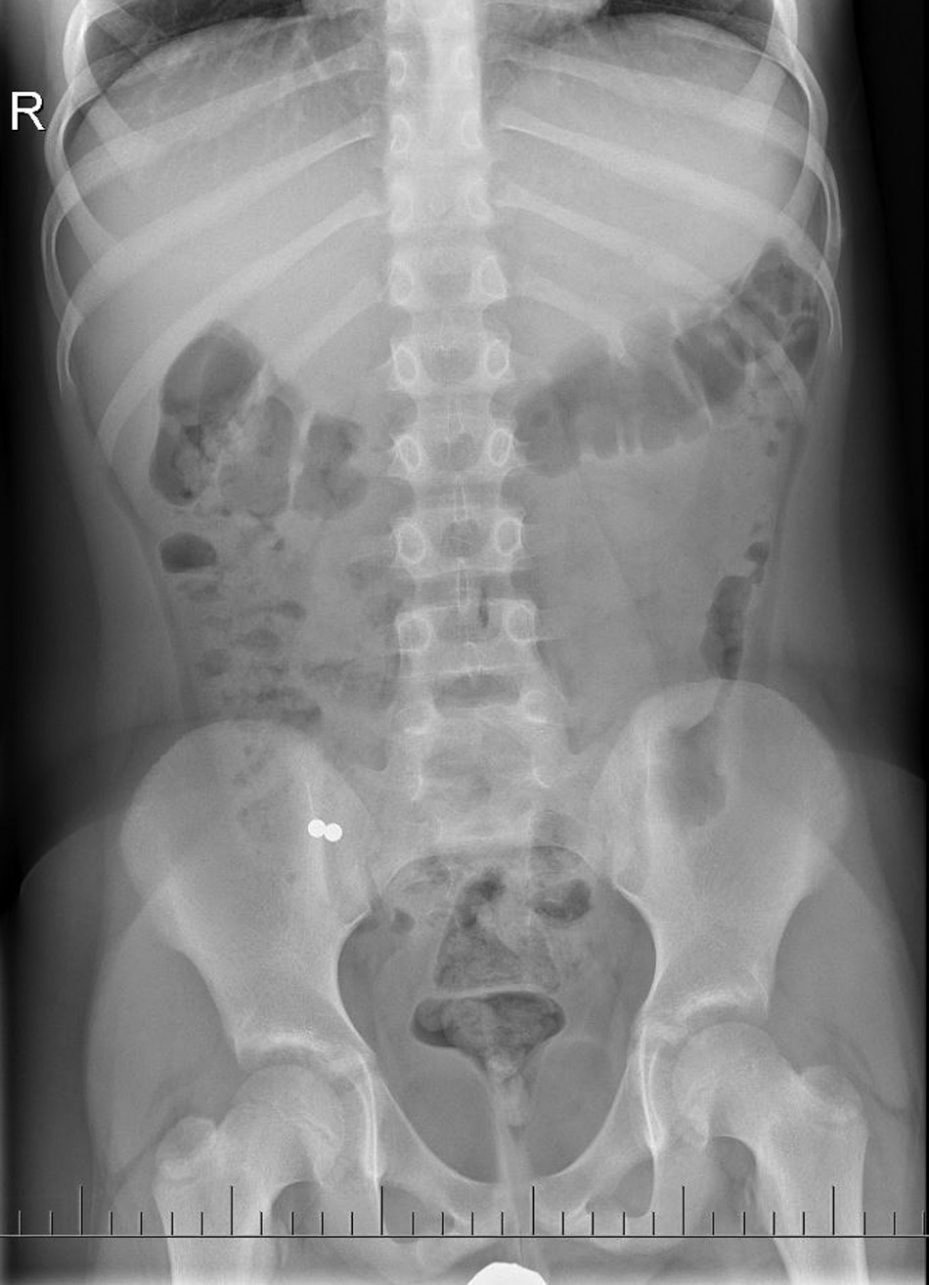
The location of MFBs on an abdominal X-ray native abdominal image. MFBs, magnetic foreign bodies.
